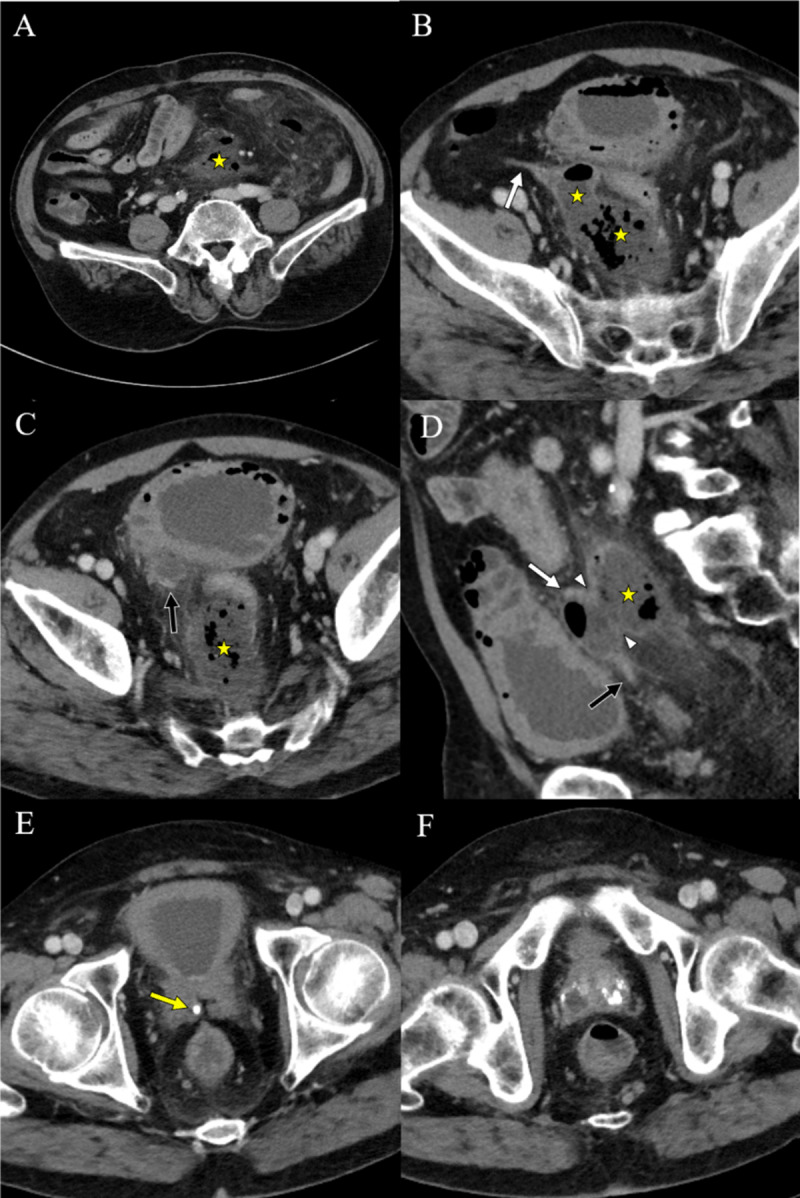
Pre-treatment CT images of a 65-year-old man with rupture of a vas deferens (VD) abscess. Axial and sagittal images (A–D) show a large abscess along the sigmoid mesocolon. Axial images (B, C) show the proximal and distal portion of right VD. A sagittal image (D) shows a wall defect of the VD adjacent to the abscess. An axial image (E) shows a tiny stone at the point where the VD joins the seminal vesicle. Axial images (F) show multifocal abscesses and dystrophic calcifications in the prostate. (asterisk: abscess, white arrow: proximal VD, black arrow: distal VD, arrowhead: VD wall defect, yellow arrow: VD stone).
